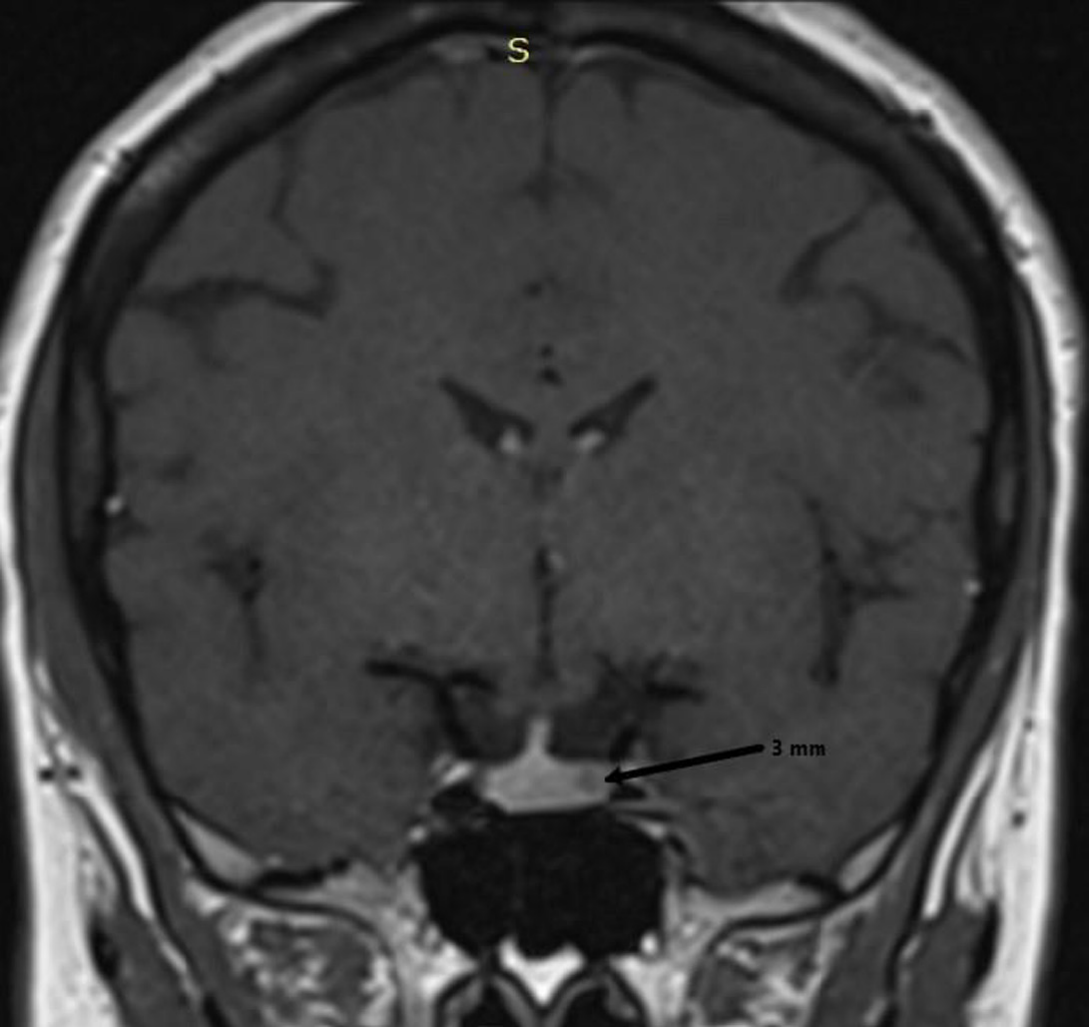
Pituitary MRI with gadolinium contrast, coronal T1‐weighted image showing a 3‐mm picoadenoma on the left side of the pituitary.
